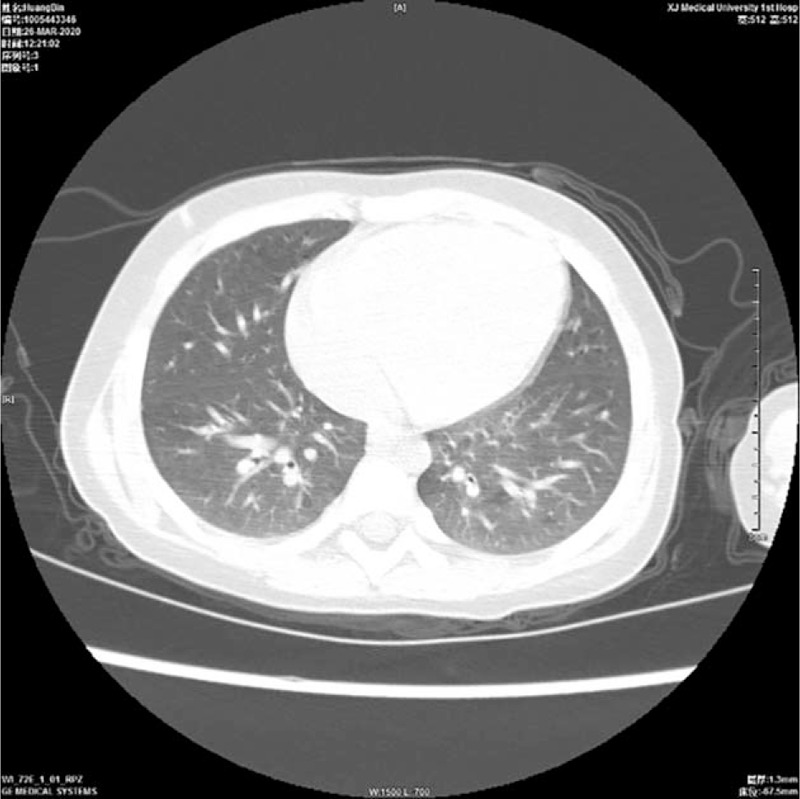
Lung computed tomography indicated that multiple granulomas were present.
